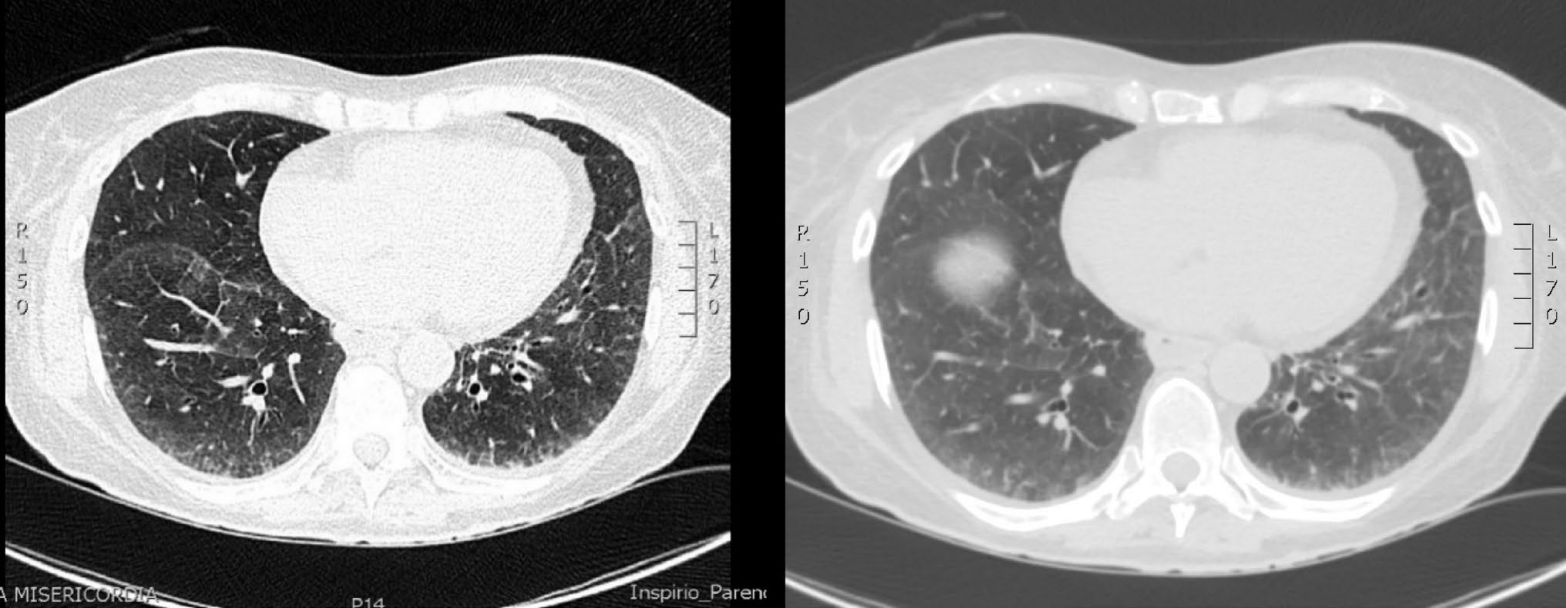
HRCT showing a mosaic attenuation pattern and diffuse ground-glass opacities at the lung bases with interlobular septal thickening
